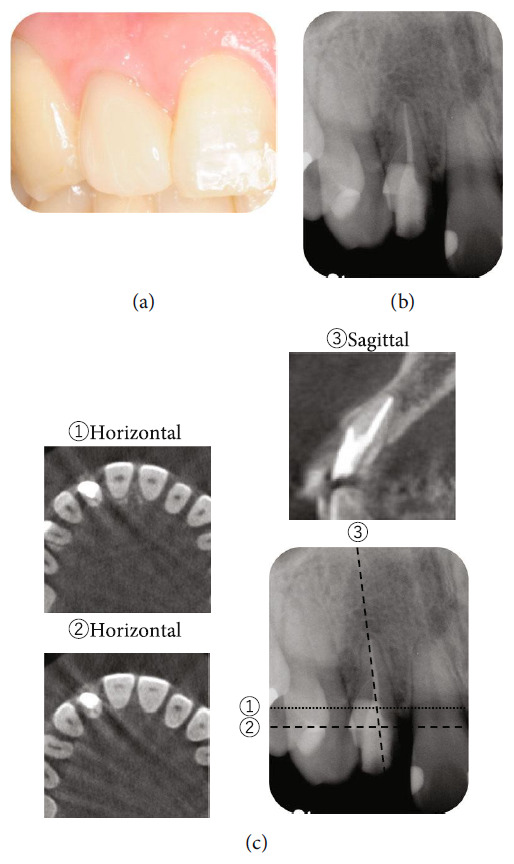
Follow-up visit 18 months after intentional replantation. (a) Intraoral photograph was taken 18 months after intentional replantation. No gingival redness or swelling was observed. (b) Intraoral radiograph was taken 18 months after intentional replantation, with no evidence of recurrence of ECR or secondary root resorption after intentional replantation. (c) Representative examples of horizontal and sagittal images of CBCT volumes; 3D evaluation by CBCT showed that the ECR lesion was now filled by the restorative material, and good healing was observed. The numbers in the figure indicate the cross-sectional view of each line of that number.
